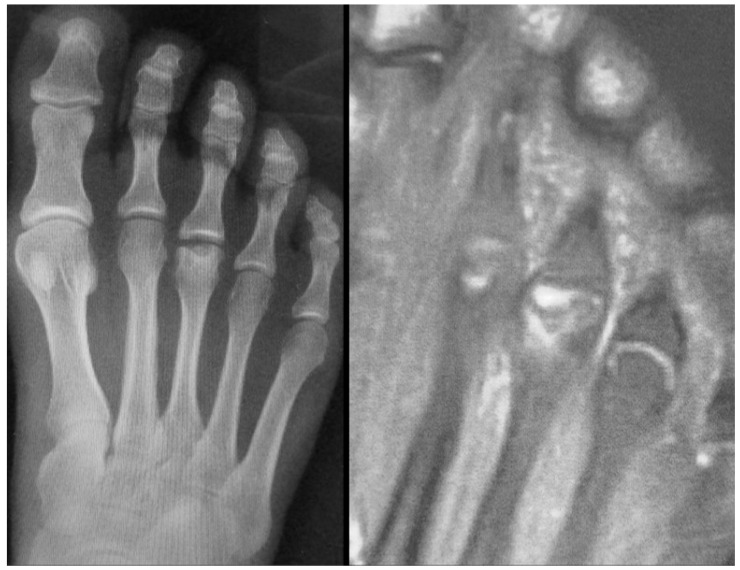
Radiograph (left) and MRI (right) of a 15-year-old male patient with osteochondrosis of the third metatarsal head and early signs of deformation.
